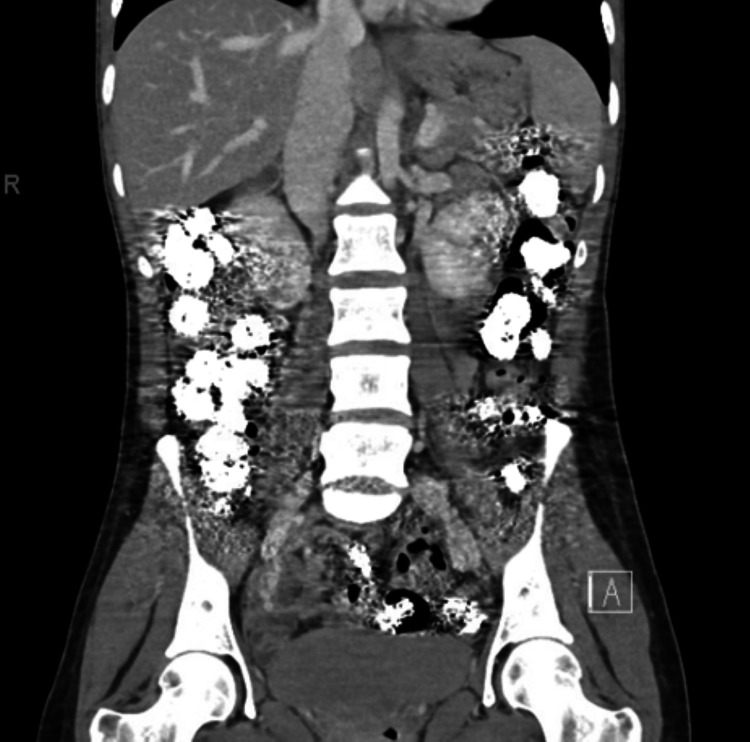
Corneal view CT of the abdomen and pelvis demonstrating hyperdense material
